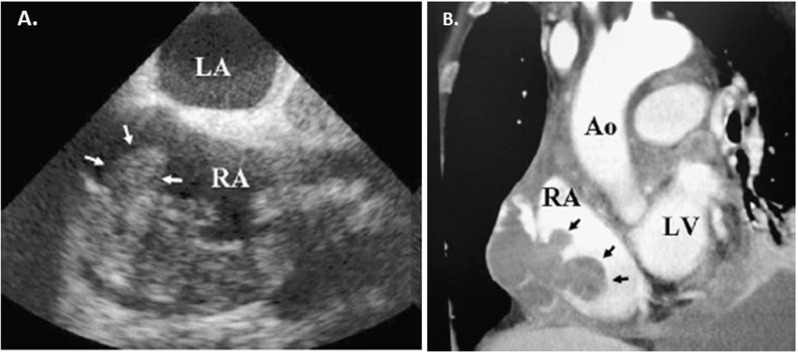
Angiosarcoma. a. Transesophageal echocardiogram demonstrating a large, lobulated mass in the right atrium. b. Contrast non-gated cardiac CT demonstrating a hypodense, multilobular mass in the right atrium. The mass invaded into the pericardial space. Aorta (Ao), Left atrium (LA), Left ventricle (LV), Right atrium (RA)
